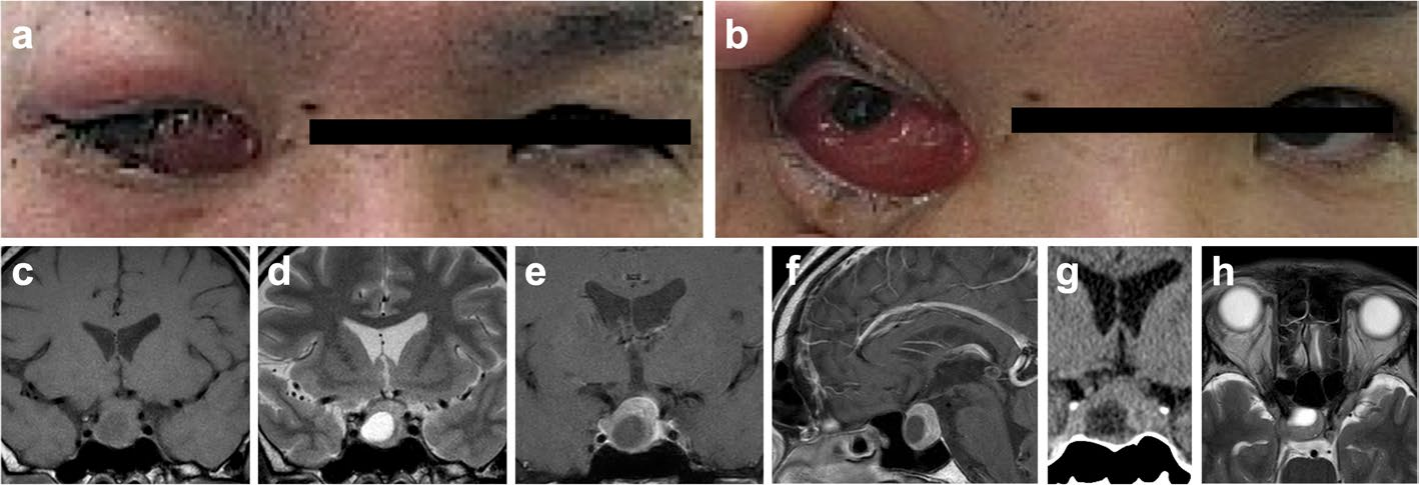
a, b Preoperative photographs of the patient’s face demonstrating marked right eye conjunctival chemosis (a: opening by self, b: opening manually). c–f Findings of initial magnetic resonance images (c: coronal T1-weighted imaging, d: coronal T2-weighted imaging, e: coronal gadolinium-enhanced T1-weighted imaging, f: sagittal gadolinium-enhanced T1-weighted imaging). g Findings of initial coronal computed tomography scan. h Findings of initial axial T2-weighted magnetic resonance image focusing around the orbits
